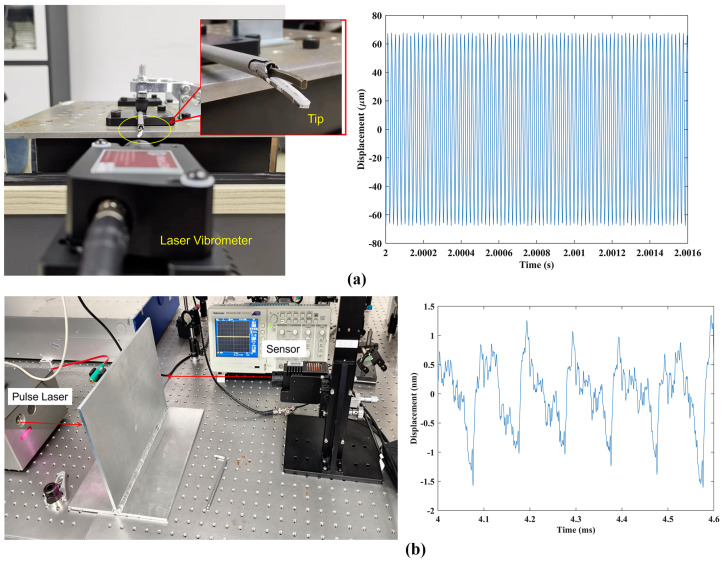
(a) Vibration of a medical scalpel tip; and (b) the measurement of ultrasonic waves induced by a high-energy laser pulse.
